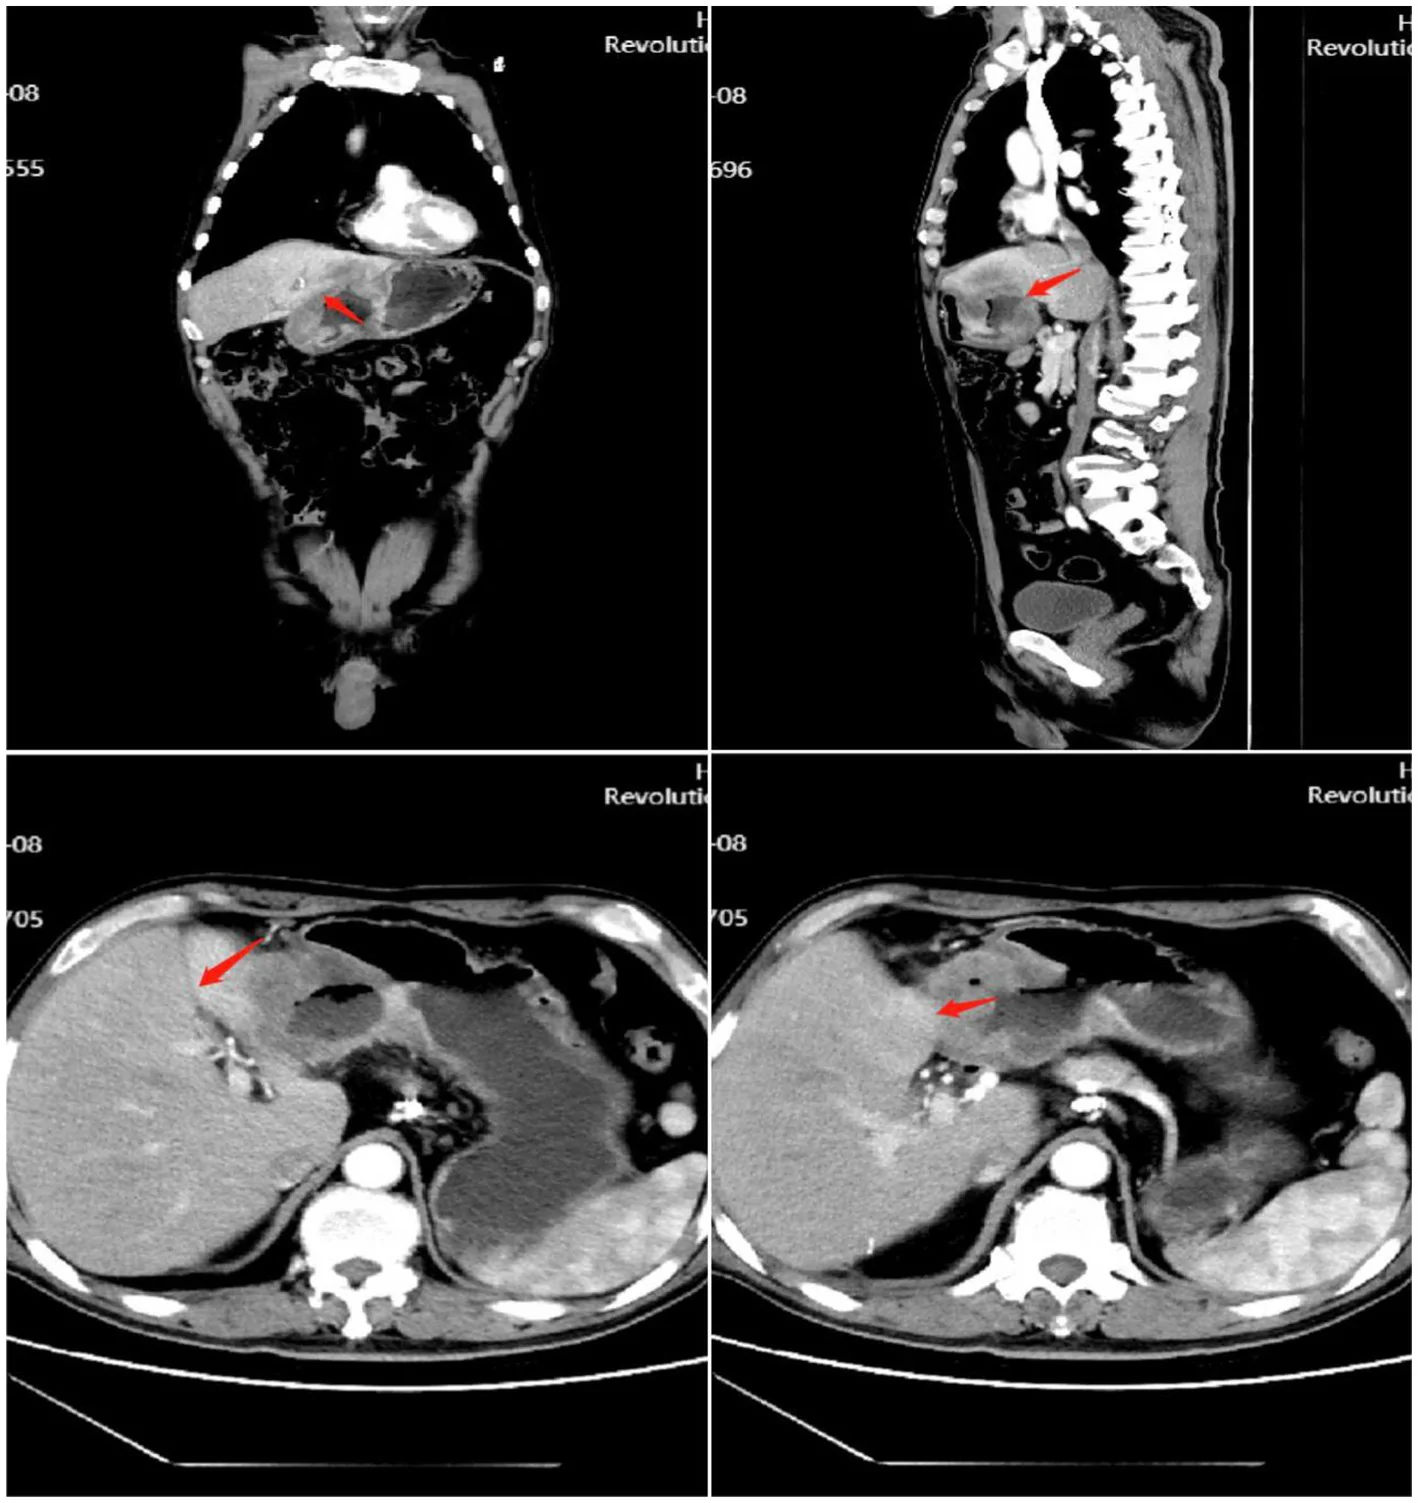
This is a high-resolution CT scan of the abdomen, showing coronal, sagittal, and transverse views; the red arrows indicate the gastric cancer invading the transplanted liver.
